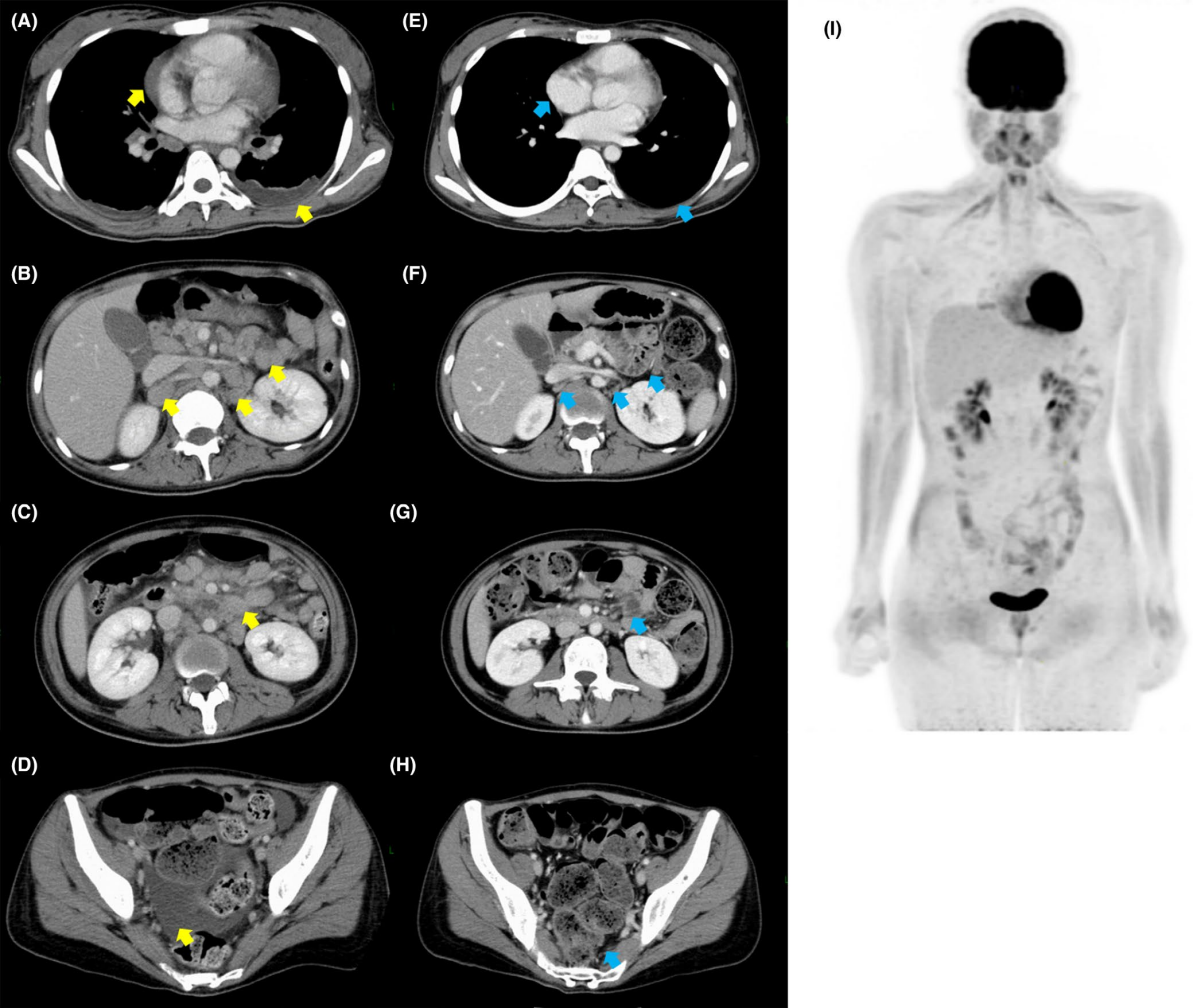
A‐D, CT images before treatment with alectinib show bilateral pleural effusion, pericardial effusion, ascites, and multiple lymph node enlargement (yellow arrows). E‐H, CT images after treatment with alectinib (day 12) show disappearance of bilateral pleural effusion, pericardial effusion, ascites, and multiple lymph node enlargement (blue arrows). I, FDG‐PET/MRI images after treatment with alectinib (day 24) show no abnormal uptake
